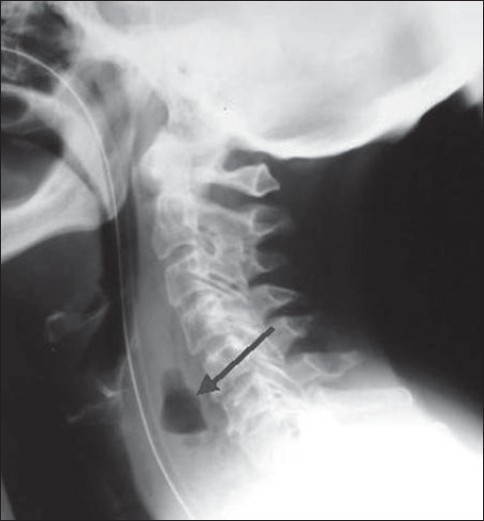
A lateral radiograph of the neck showing a collection of air in the prevertebral soft tissue space due to esophageal perforation secondary to a difficult intubation (arrow)
